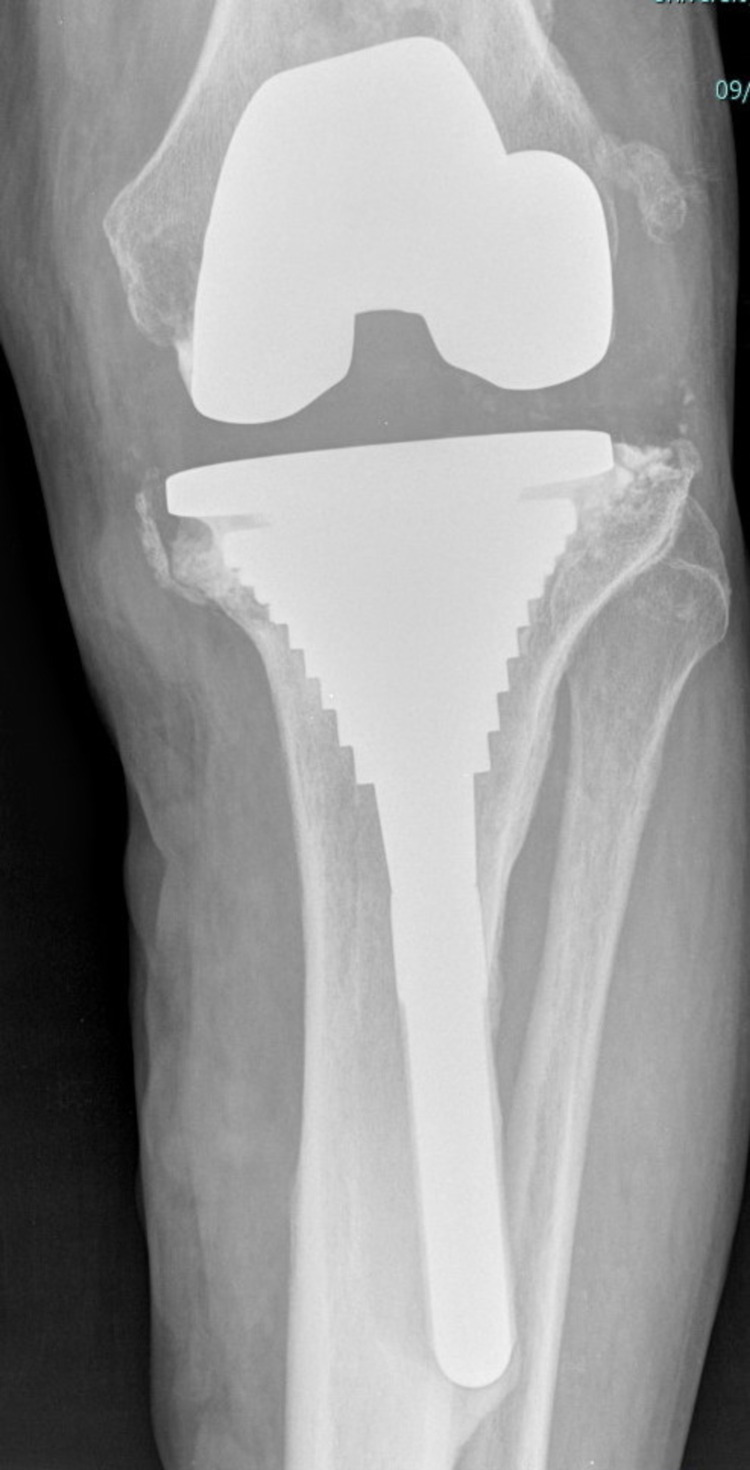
A 72-month follow-up X-ray anteroposterior view. The tibial component has loosened and migrated into varus.
